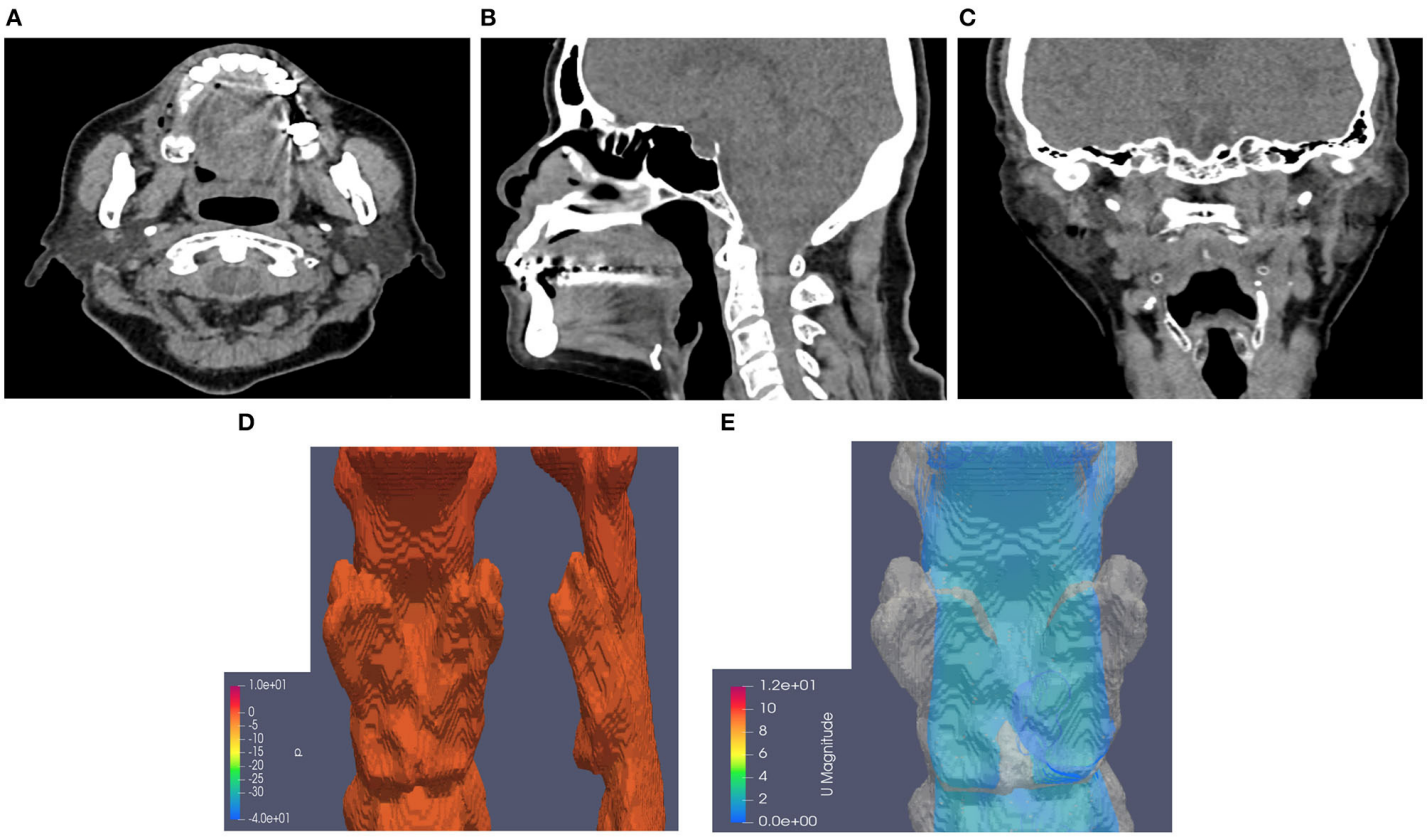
Neck CT with computational fluid dynamics study of the 82-year-old female showed CTL12. (A) Axial view. (B) Sagittal view. (C) Coronal view. (D) Airway pressure of 1.28 Pa. (E) Airflow velocity of 0.8 m/s.
